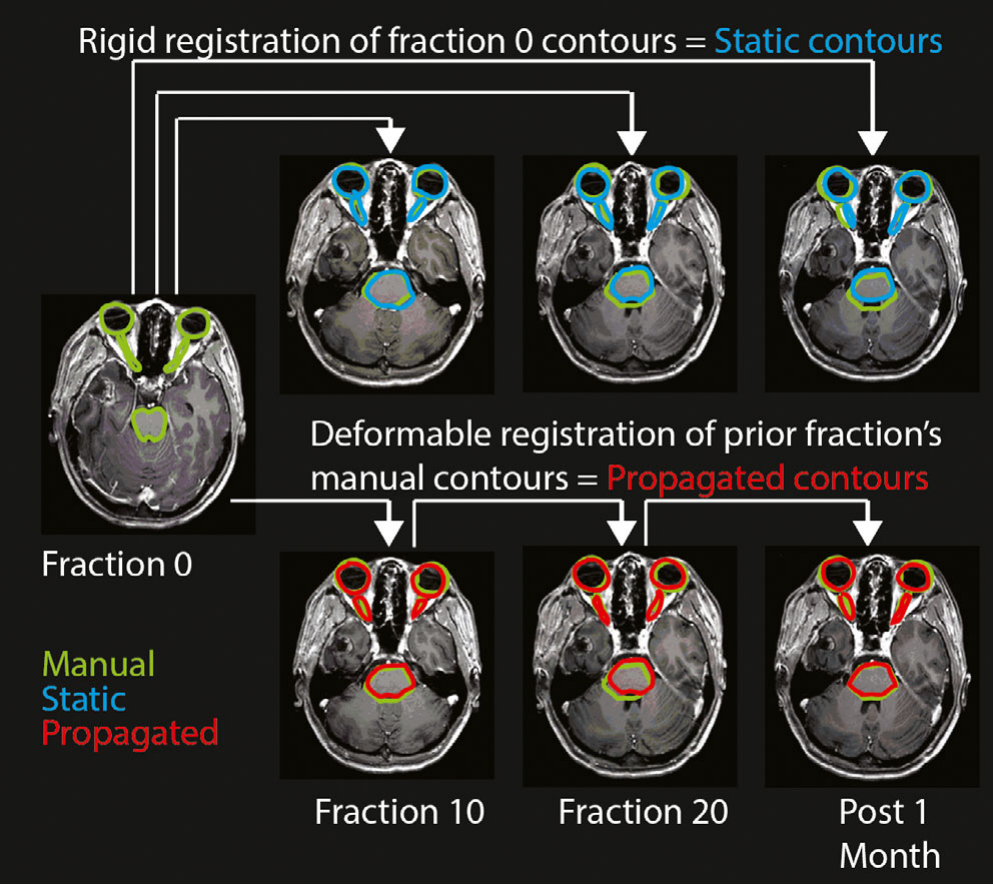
Static contours and propagated contours compared to manual contours. Manual contours (green) generated on each time point MRI were compared to static contours (blue) and to propagated contours (red). Static contours were the fraction 0 manual contours rigidly registered onto the MRI of the current fraction. Propagated contours were deformably registered from the manual contours of the prior time point. Arrows connect the baseline atlas to the resulting segmentation.
